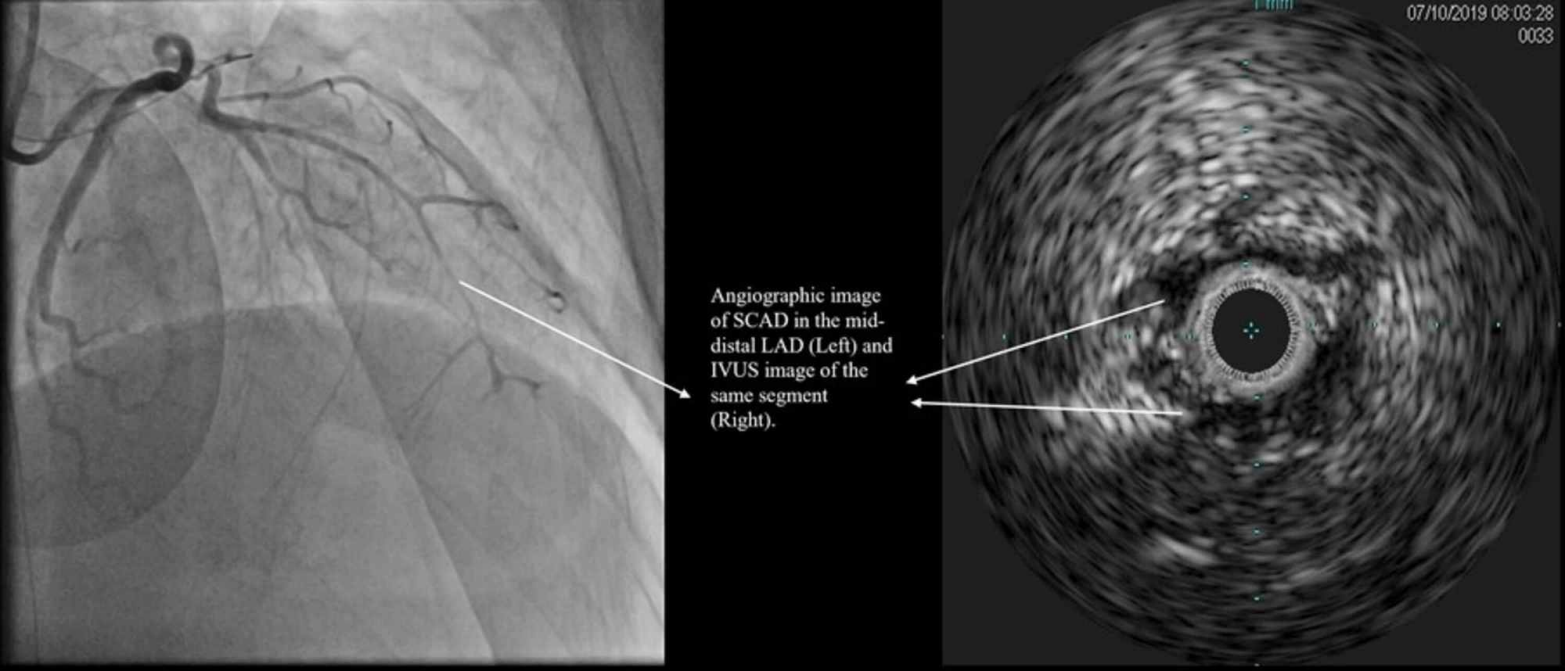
Spontaneous coronary artery dissection of the mid-distal left anterior descending artery SCAD: Spontaneous coronary artery dissection; LAD: Left anterior descending; IVUS: Intravascular ultrasound.
